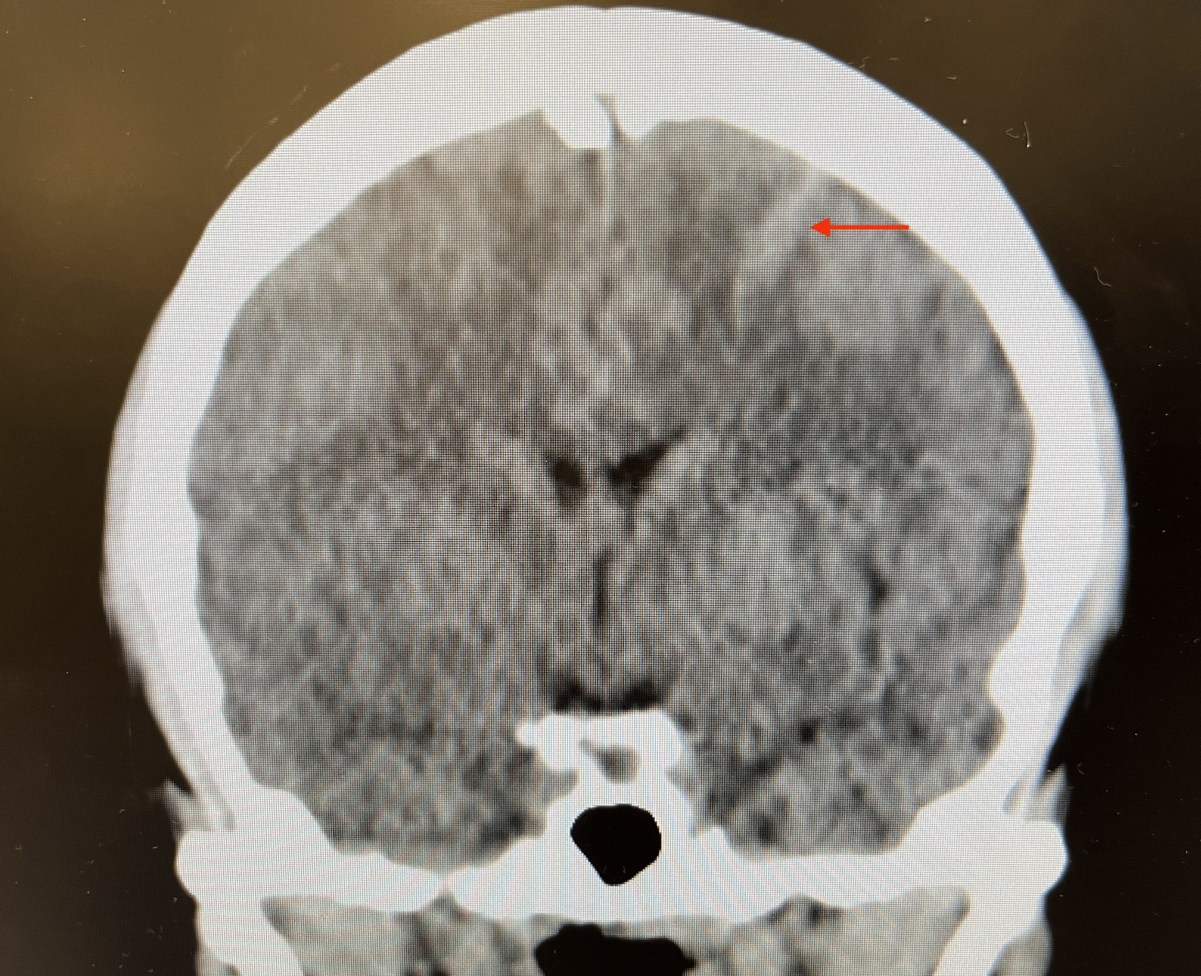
CT scan of the brain without contrast showing a left-sided subarachnoid hemorrhage suspicious in this setting for a possible mycotic aneurysm.
